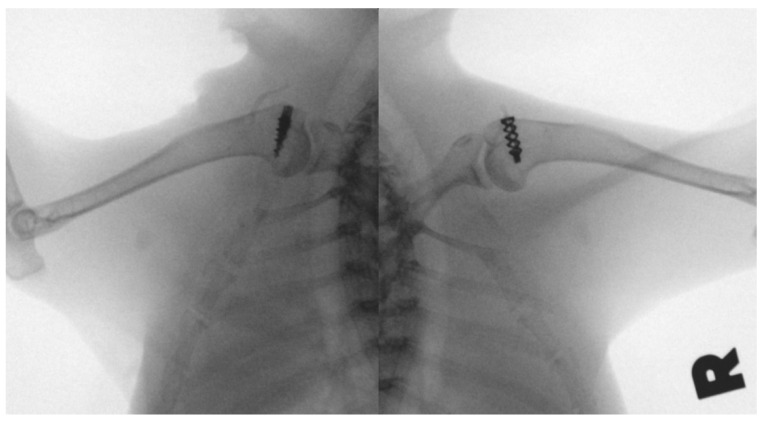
The radiograph shows that the Ti SA (left shoulder) and Fe SA (right shoulder) were inserted into the proximal humerus greater tuberosity.
